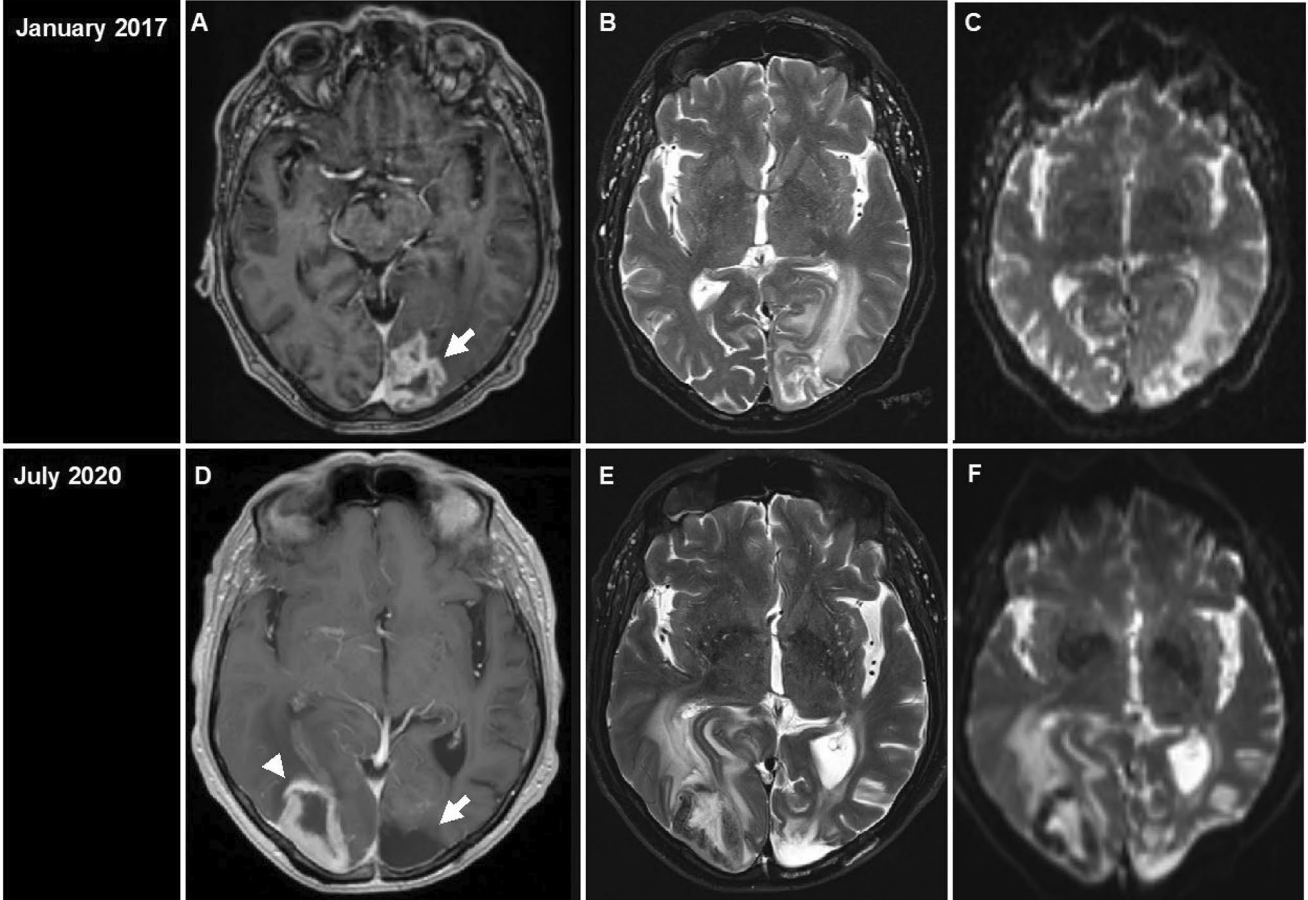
Post-gadolinium magnetic resonance imaging (MRI). T1 gadolinium-enhanced MRI from January 2017 (A–C) and July 2020 (D–F) showed abscess-like lesion with involvement of the left occipital lobe in January 2017 (A, white arrow) and right occipital lobe in 2020 (D, white arrowhead). Note the old, cyst-like cortical defect in the left occipital lobe in 2020 (D, white arrow), vasogenic edema and mass effect with displacement of the temporal horn of the right lateral ventricle
